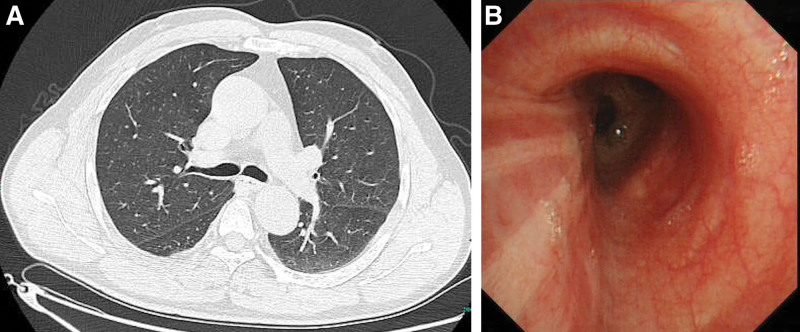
Follow-up examinations findings. (A) The left upper main bronchus was clear and no evidence of recurrence of neurofibroma on chest CT. (B) Repeated bronchoscopy showed no regrowth of the neurofibroma, and the procedure site was healed with a little of fibrotic scar formation. CT = computed tomography.
